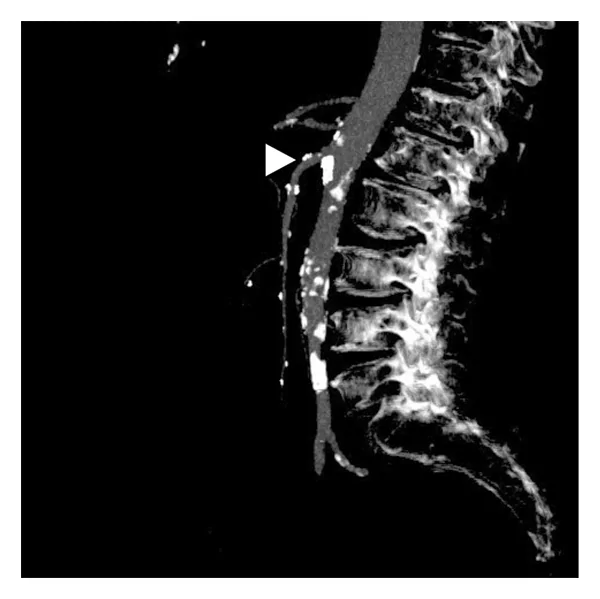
Sagittal maximum intensity projection (MIP) reformats of the abdominal CT angiography display calcific plaques in both the proximal segment of the SMA (arrowhead) and the abdominal aorta. These calcifications appear as bright spots, with the sagittal view providing a longitudinal section that highlights the extent of plaque deposition along the vessel walls.
